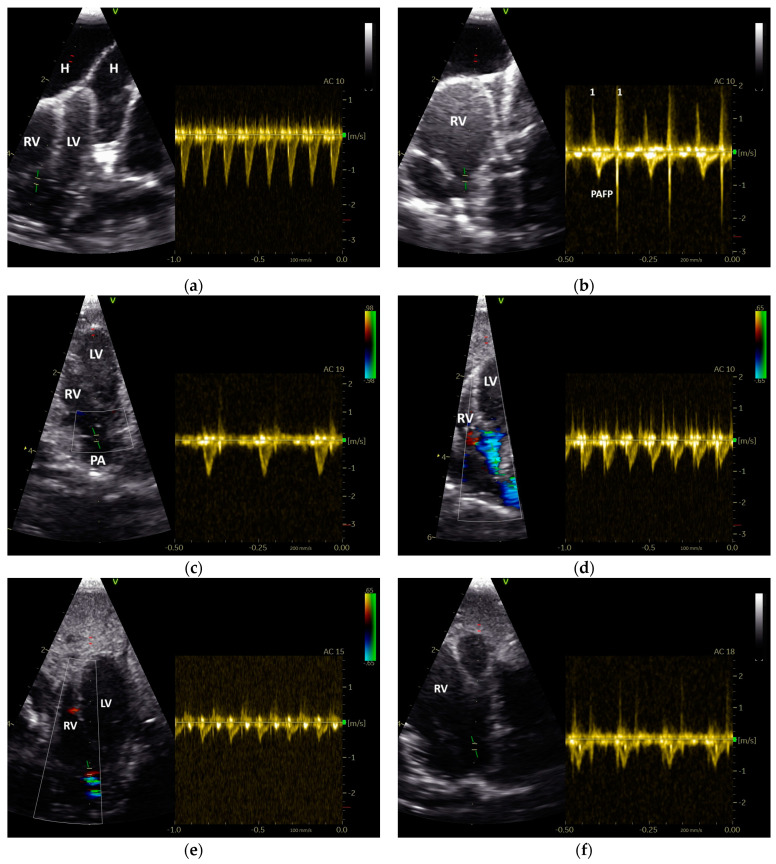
PW Doppler sonographic blood flow profiles of the pulmonary artery of Congo grey parrots with left and right heart failure ((a–f); GPs from group 3). In all presented cases, the abnormally short acceleration phase (AP) of the pulmonary blood flow profile (PAFP) is visible; RV: right ventricle; LV: left ventricle; PA: pulmonary artery; H: hepatoperitoneal cavity effusion; 1: Doppler signal of the pulmonary valve, with a sample gate placed in the area of the pulmonary valve; AC: angle correction. The colour scale on the right side of the images is calibrated in m s^−1^.
